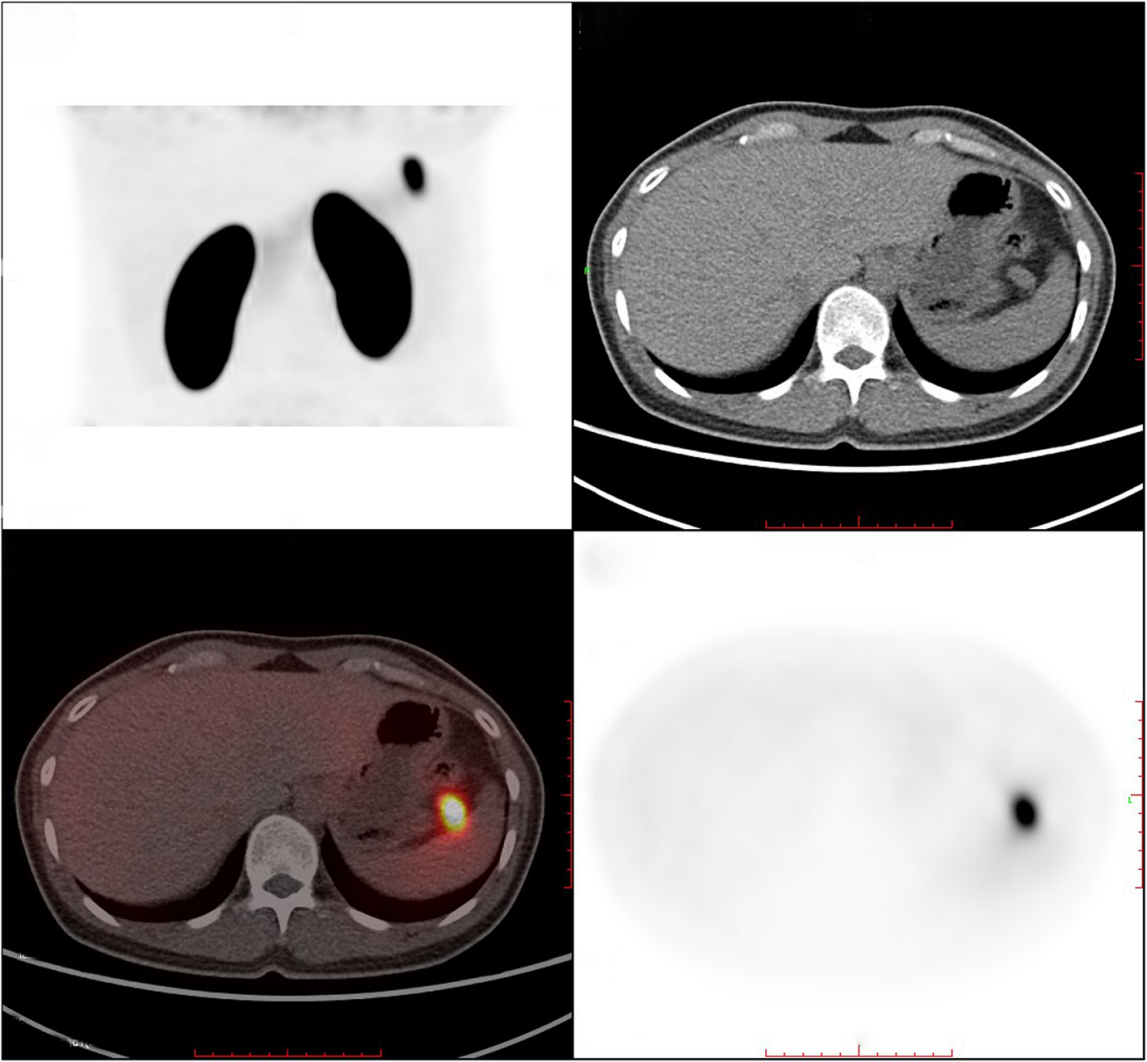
Case 2: 68Ga-Exendin-4 PET/CT showing intense uptake in the nodule near the splenic hilum, suggesting an insulinoma
